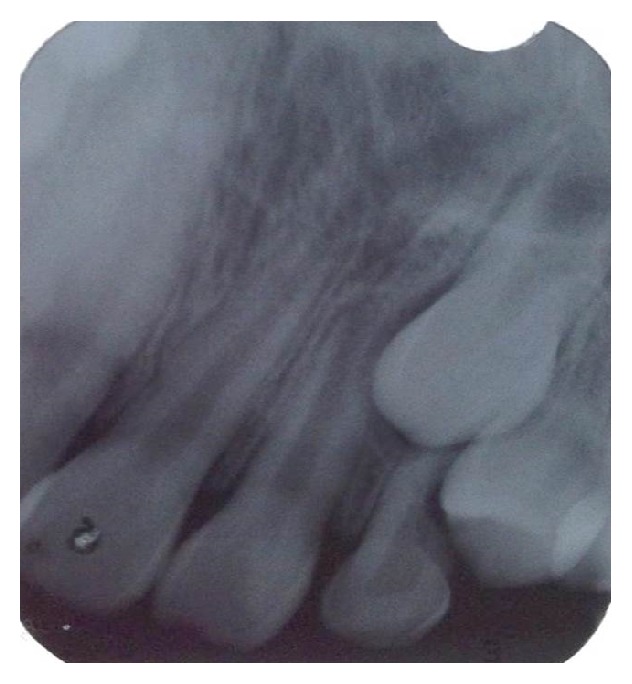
Radiographic aspect of the lesion without signs of abnormality.
